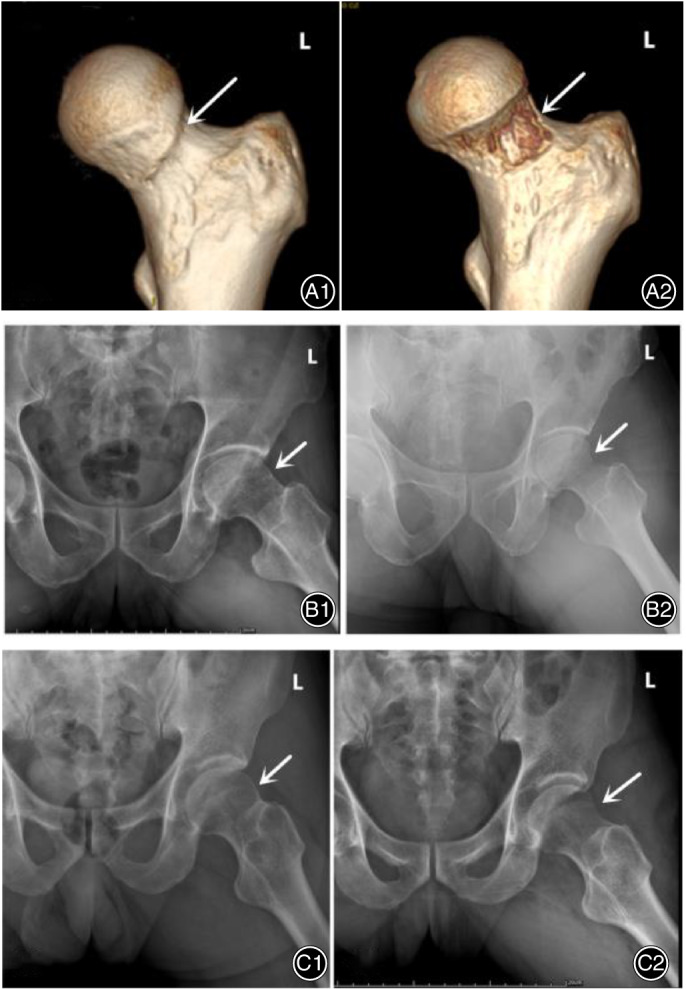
Preoperative CT (A1) and X‐rays (B1, C1) of femoroacetabular impingement (FAI) in 3 patients (A, 54‐year‐old woman; B, 58‐year‐old man; C, 59‐year‐old man); postoperative CT (A2) and X‐rays (B2, C2) of arthroscopy for FAI (arrows indicate changes before and after surgery).
